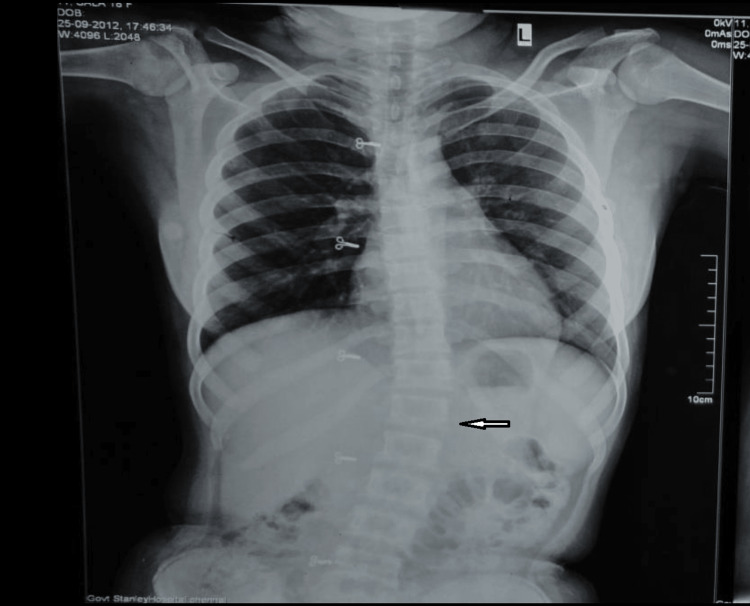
X-ray chest and spine (PA view) shows kyphoscoliosis at T10-L2 vertebral levels (arrow). PA: posteroanterior
